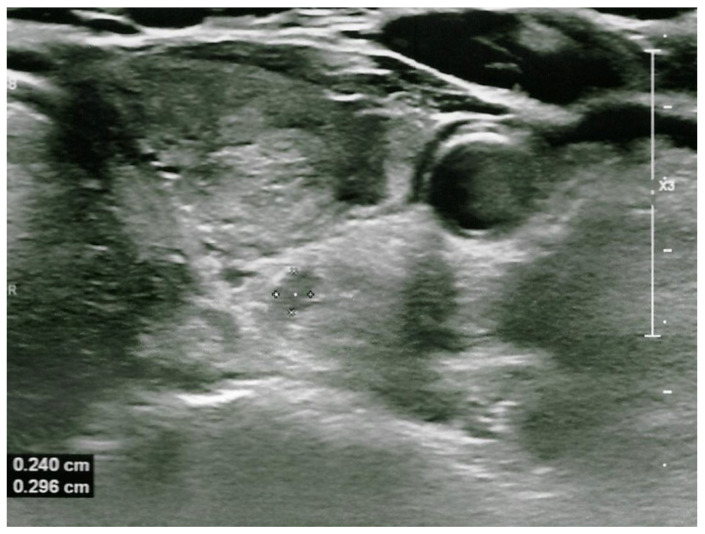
The ultrasonography demonstrated a marked reduction in the size of the previously identified parathyroid adenoma, now measuring 2.4 × 2.9 mm, following the second fine-needle aspiration biopsy (FNAB).
